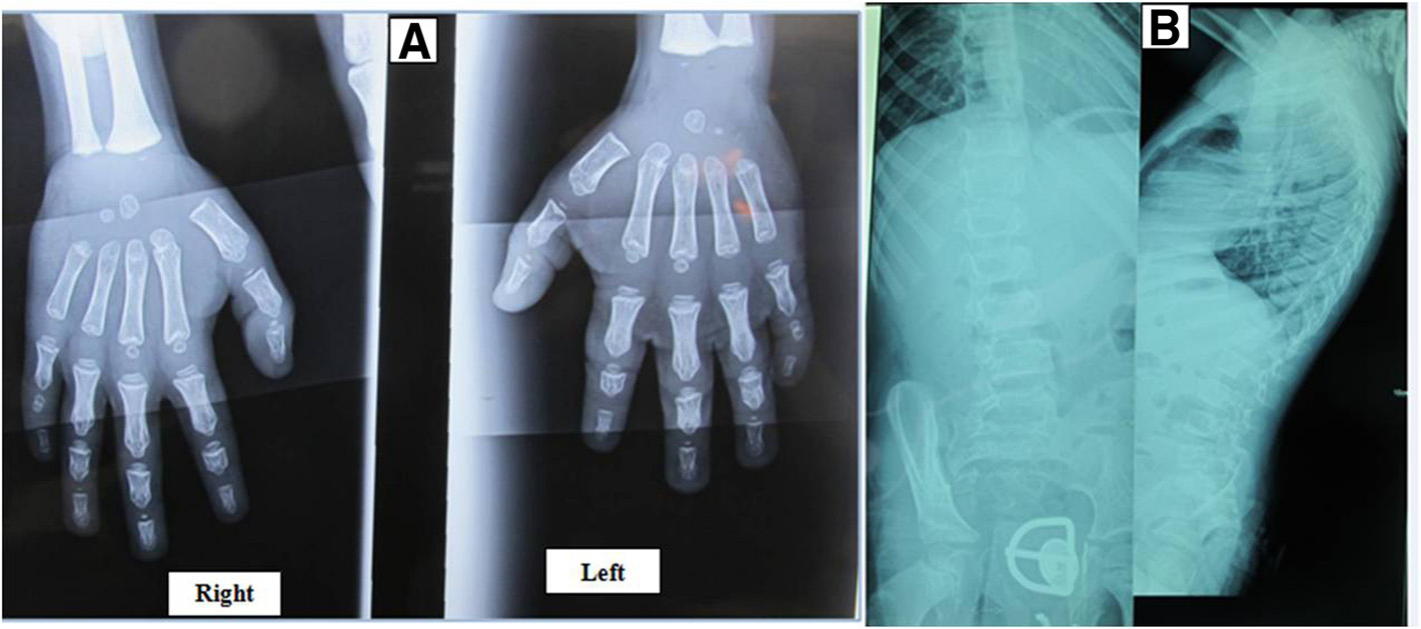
Hands-wrist and lumbar spine radiographs of the boy at the age of 30 months. a Hands-wrist radiograph shows normal bone structure, but bone age is delayed by 3 months for a 30-month-old child. b Lumbar spine radiograph shows a deviation of the spine in the frontal plane in the form of a thoracolumbar scoliosis with a mild platyspondyly
